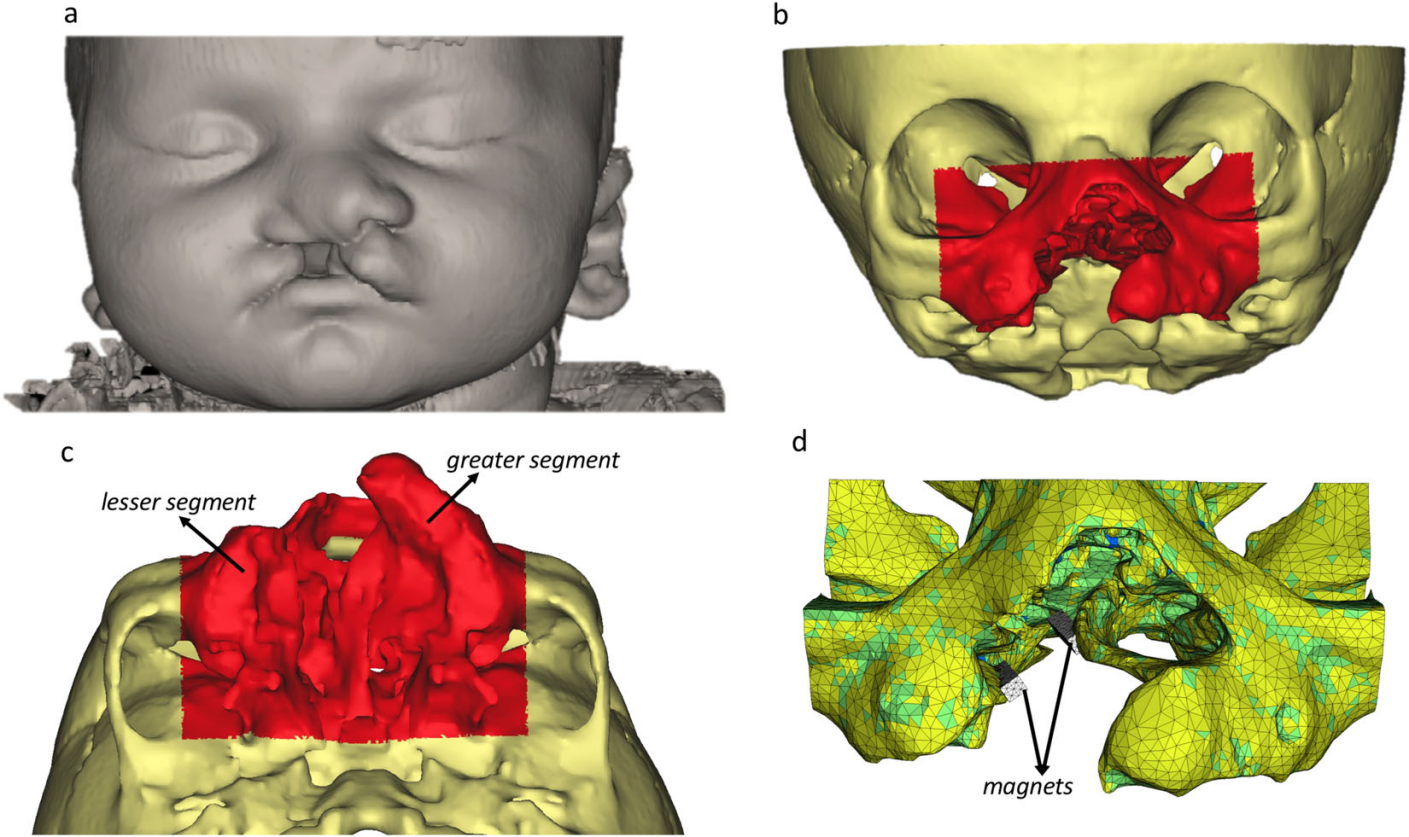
From CT scan to FE-model. a The 3D reconstructions of the soft and hard tissues, b Frontal view of the region of interest for the FE model c Palatal view of the region of interest for the FE model d and the FE model of the dental magnet partially visible
